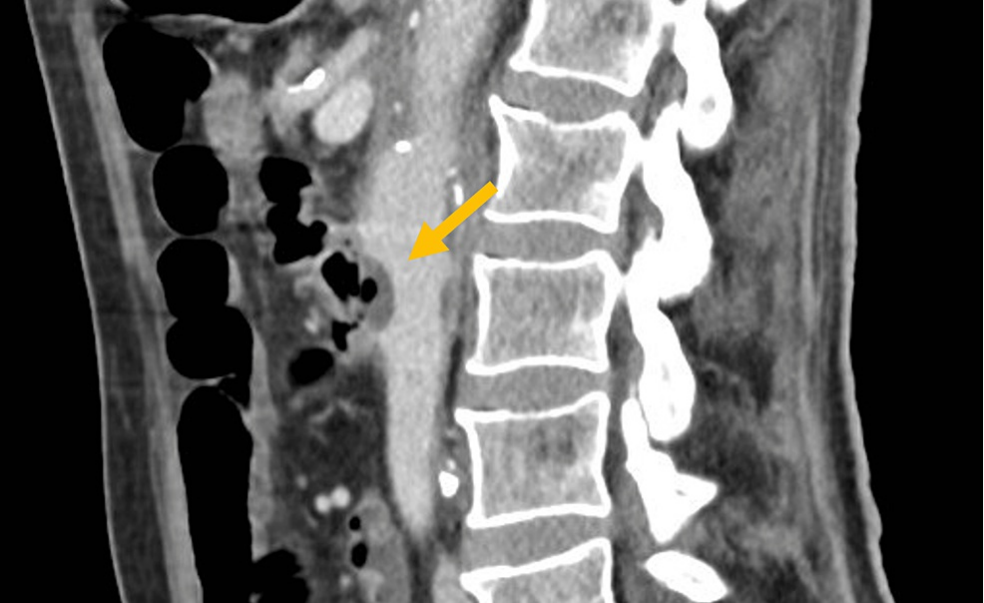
CT scan, sagittal cut (midline). Arrow pointing to gas in the aortic artery.
